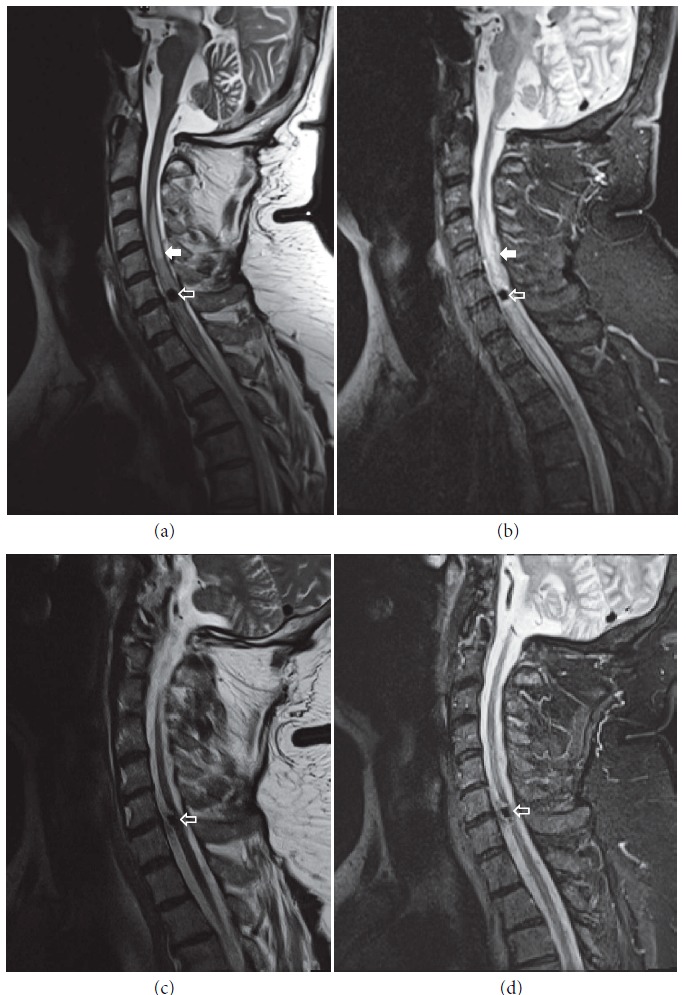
MRI of the cervical spine: initial MRI at symptom onset: (a) sagittal T2W/TSE (TR 3800 ms, TE 108 ms); (b) sagittal T2W/STIR (TR 4000 ms, TE 39 ms). Images show a hypointense oblong lesion in the spinal cord at the level of C6-C7 (empty arrows). Above and below the lesion the spinal cord shows hyperintense signal consistent with myelitis (filled arrows). Follow-up MRI 10 months later: (c) sagittal T2W/TSE (TR 3800 ms, TE 108 ms) and (d) sagittal T2W/STIR (TR 4000 ms, TE 39 ms). Follow-up images show normal spinal cord appearance following the resolution of myelitis and the same hypointense glass lesion (empty arrows).
